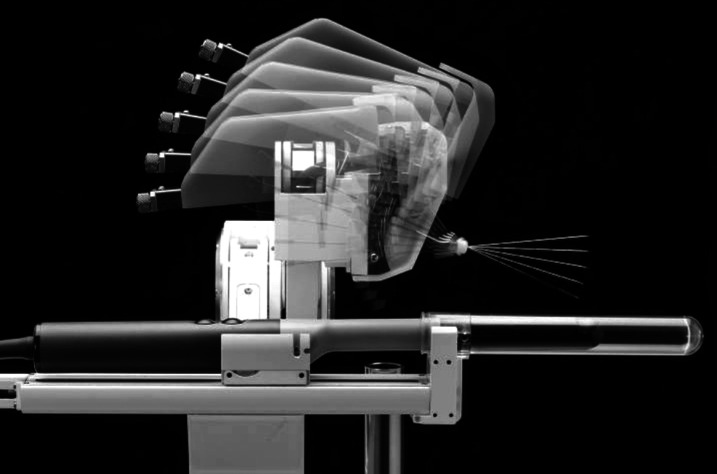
iSR’obot™ MonaLisa robotic-assisted MRI/TRUS fusion transperineal prostate biopsy system with transrectal ultrasound probe (BK 3000; BK Medical, Peabody, Mass, USA).
